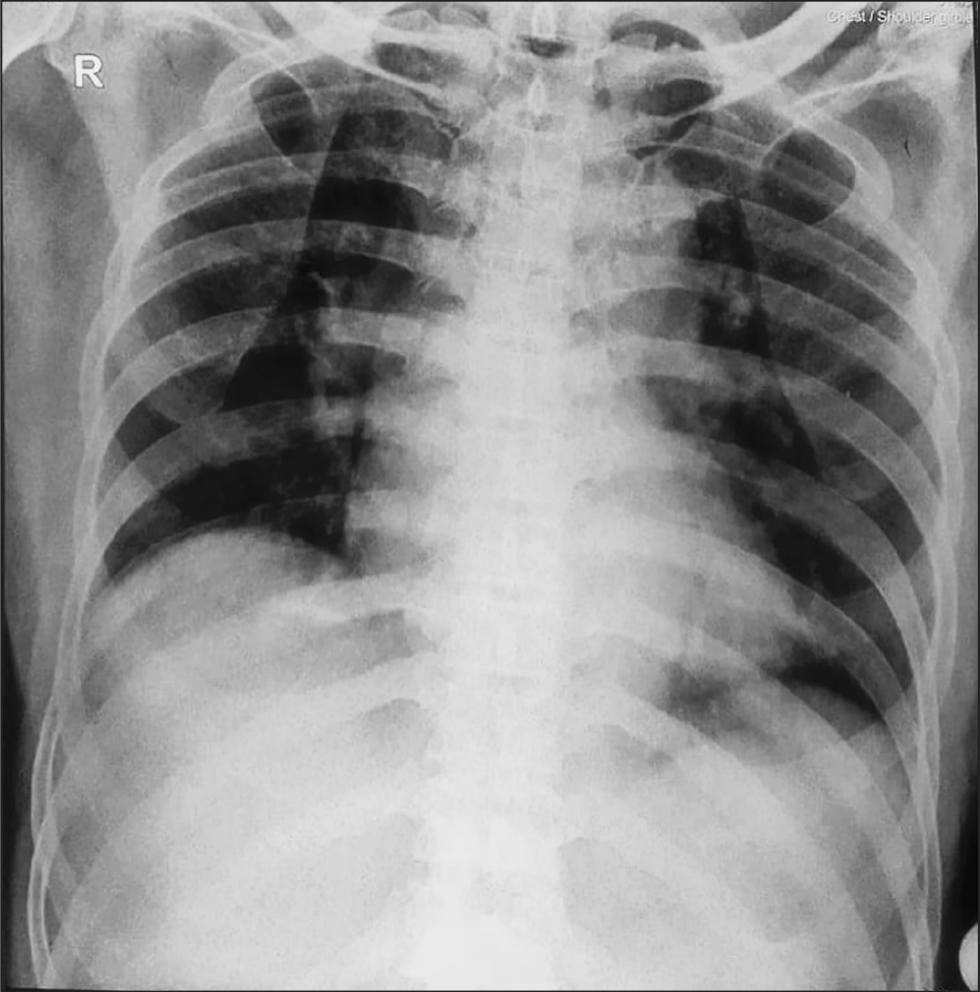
Chest radiograph at emergency department upon patient arrival. The radiograph showed clear lung fields.
